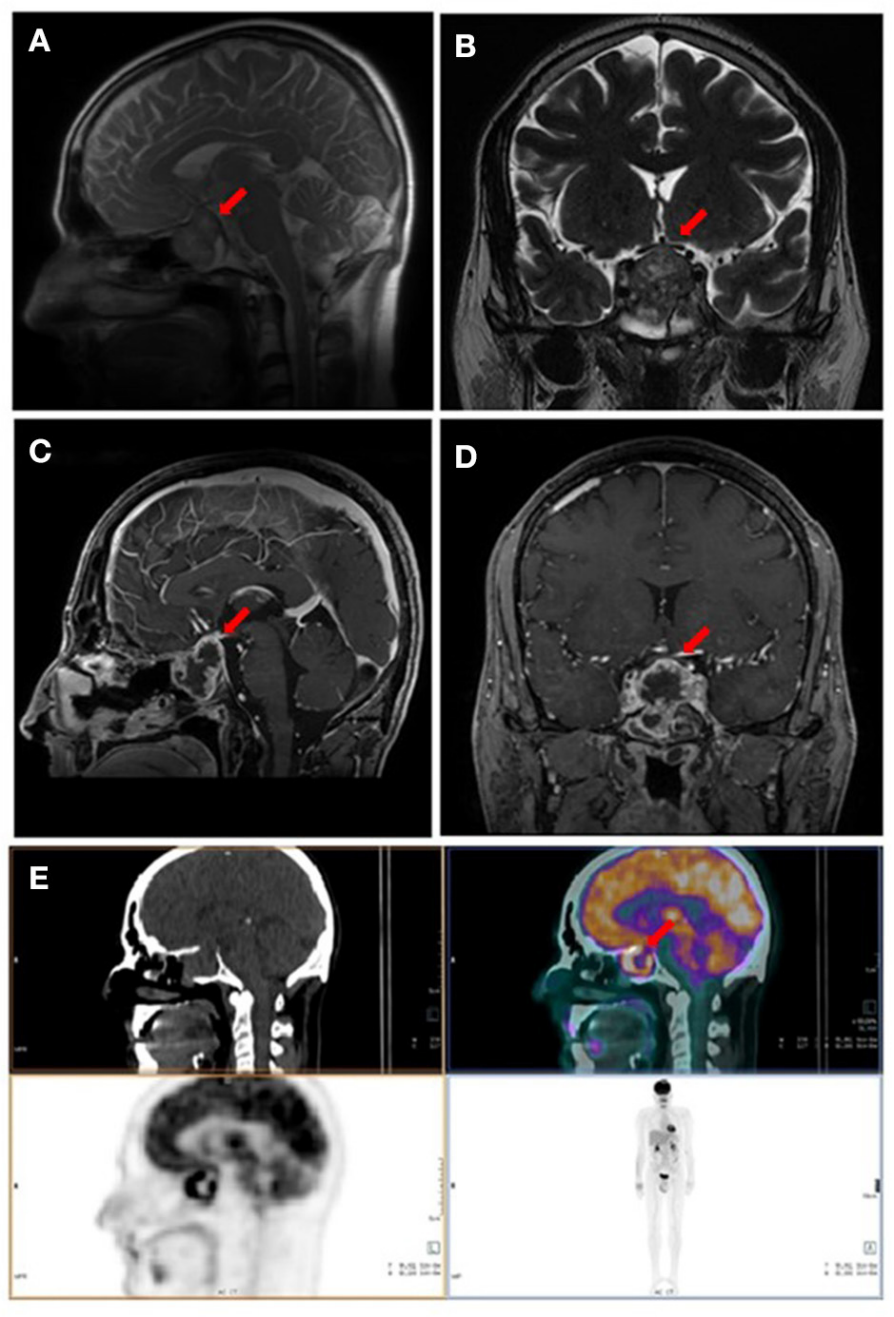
Imaging performed 3 months after surgery. (A,B) Pituitary MRI, T2-weighted basal sagittal and coronal sequences. (C,D) Pituitary MRI, T1- weighted post-gadolinium sagittal and coronal sequences. (E) Whole body fluorine-18-fluorodeoxyglucose positron emission tomography/computed tomography (^18^F-FDG PET/CT) showing pathological uptake in sellar region. Red arrows indicate the sellar lesion.
